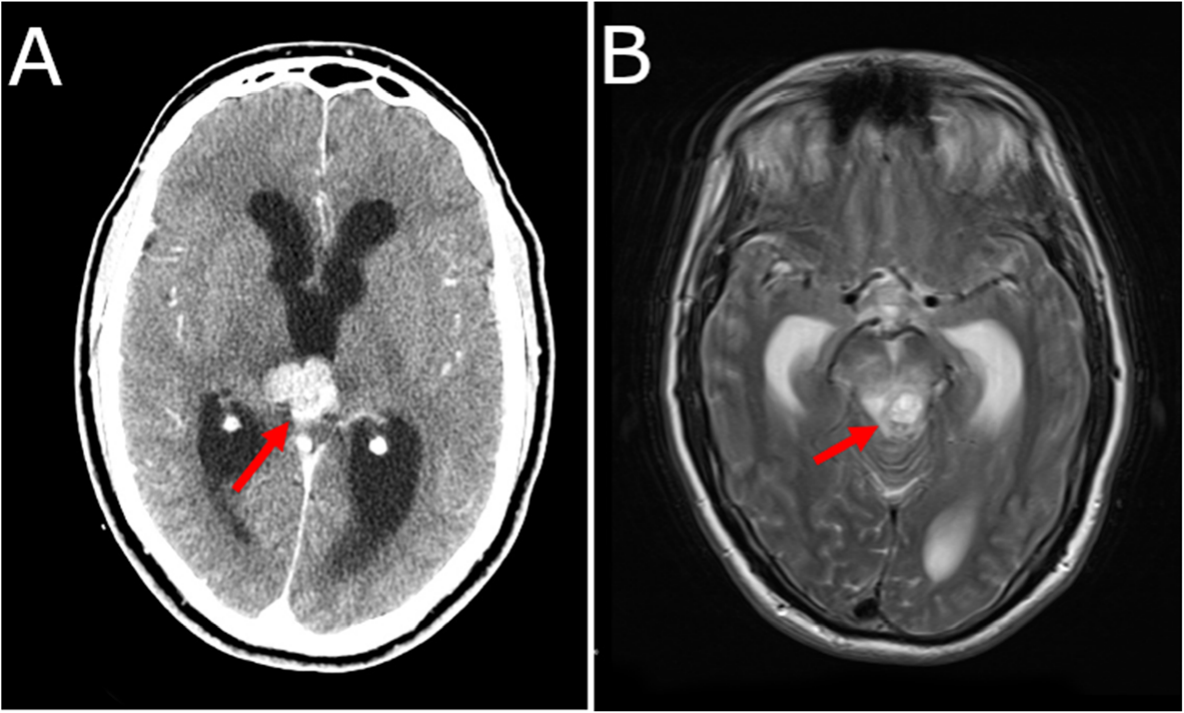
Pre-operative axial radiological images of the brain. A Computed tomography scan demonstrating the neoplasm in the pineal region with central calcification. B Magnetic resonance imaging scan demonstrating the neoplasm within the pineal region
